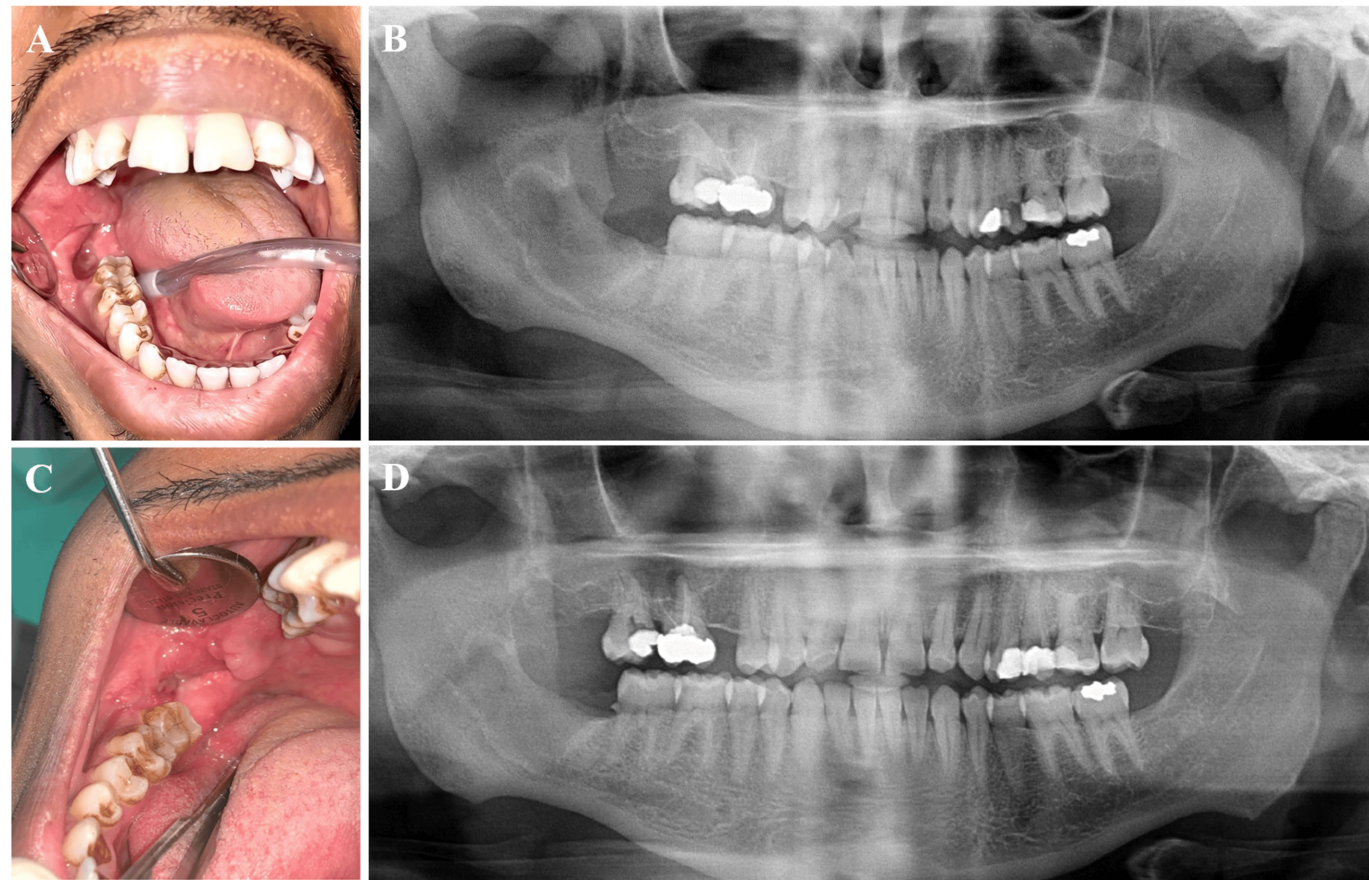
Postoperative intra-oral photographs and panoramic radiographs Two weeks of follow-up showed a clean surgical site with good initial healing (A and B). Two months of follow-up showed significant surgical site healing (C and D).
